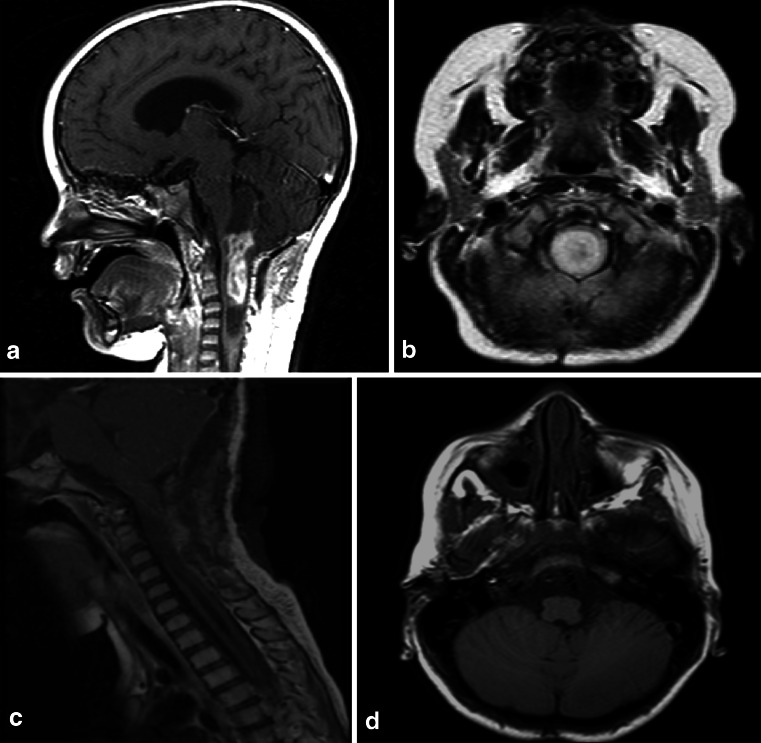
a, b Cervico-medullary tumor with exophytic extension in the fourth ventricle. c, d MRI at 5 years after surgery shows no evidence of tumor
